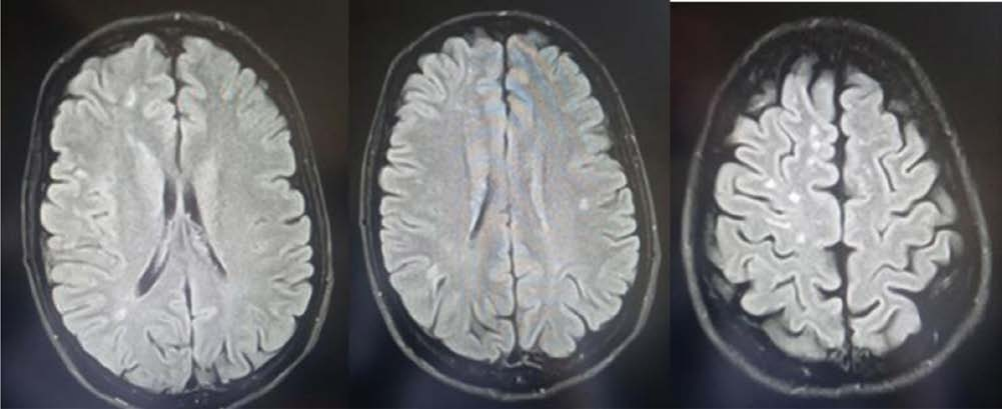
An MRI of the brain with the FLAIR technique showed multiple high-signal foci and plaques in the white matter of the hemispheres, where most of them were located in the peripheral subcortical areas. A small cavity focus was also identified in the context of an old infarction in the right posterior temporal lobe. MRI = magnetic resonance imaging.
